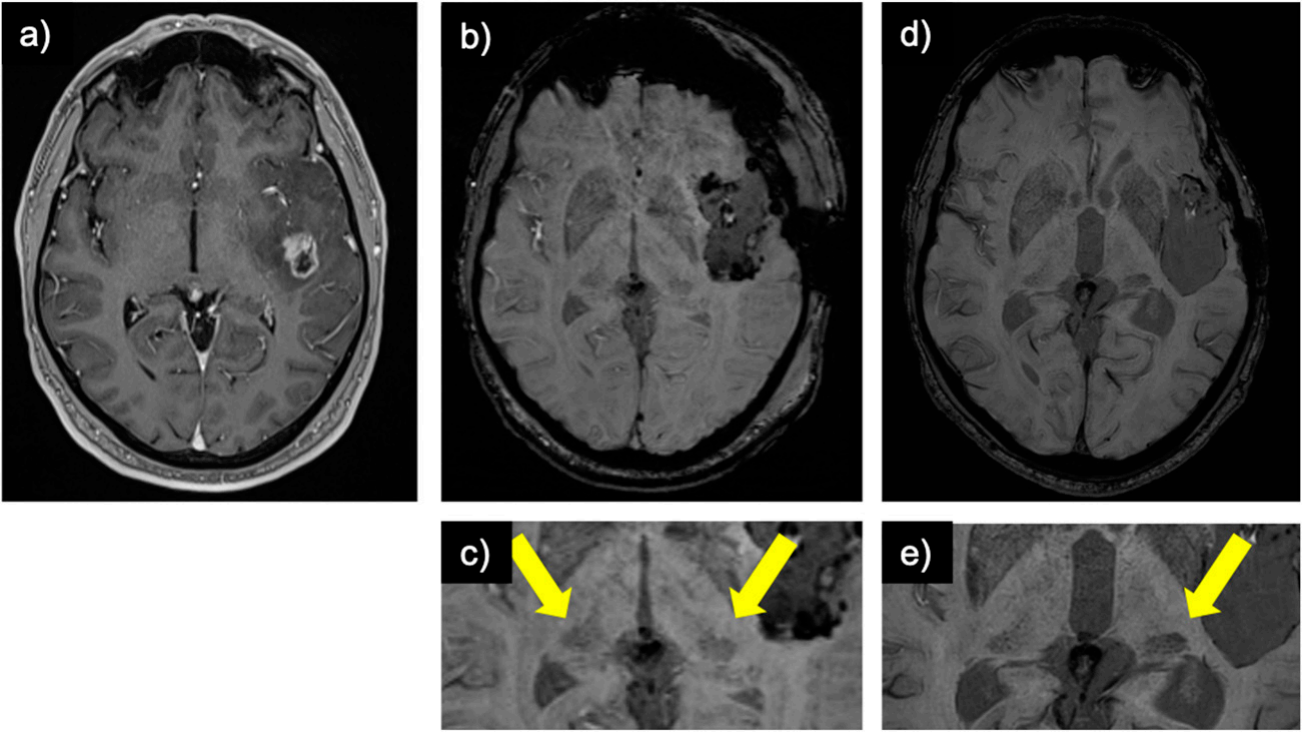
Post meningioma resection resolution of the hypointense pulvinar sign: (a) contrast-enhanced T1-weighted image of patient with a meningioma over the right parietal lobe and (b) status post-resection. (c) whole-brain susceptibility weighted image pre-resection and (d) post-resection. (e) zoomed-in susceptibility weighted image pre-resection demonstrating a right-sided hypointense pulvinar sign (arrow) and (f) resolution of the sign (i.e., symmetrical signal of the pulvinar bilaterally or “equal”) post-resection.
